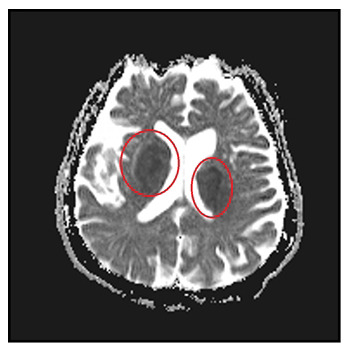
Hypointense areas consistent with diffusion-limiting ischemia in bilateral suprathalamic regions on diffusion-weighted MRI ADC sequence.
